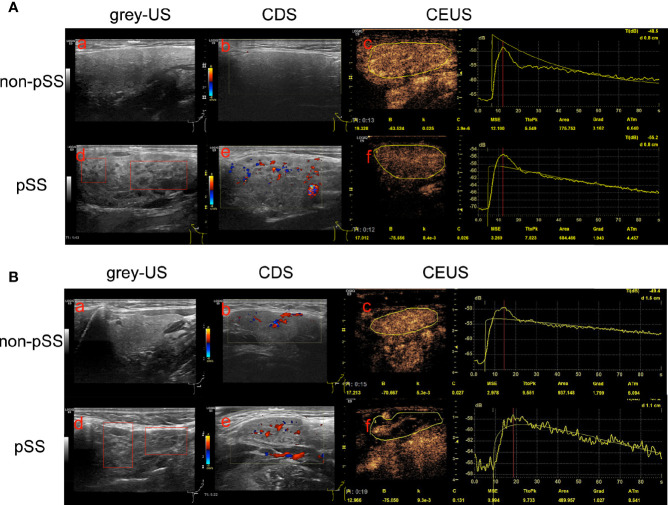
Multimodal ultrasonographic imaging characteristics of the parotid and submandibular glands between the primary Sjögren’s syndrome (pSS) and non-pSS subgroups. (A) Parotid glands: (a) homogeneous parenchyma without anechoic or hypoechoic areas in the non-pSS cohort; (d) diffuse inhomogeneity with anechoic or hypoechoic areas in pSS patients; CDS identified abundant hypervascularization in pSS cohorts (e) with poor vascularization in non-pSS groups (b); (c, f) No echo-free areas and delay TtoP in the parotid glands of pSS compared with non-pSS cohorts. (B) Submandibular glands: (a) homogeneous parenchyma without anechoic or hypoechoic areas in the non-pSS cohort; (d) diffuse inhomogeneity with anechoic or hypoechoic areas in pSS patients; (b, e) abundant hypervascularization detected in both pSS and non-pSS patients; (c, f) massive echo-free areas without contrast enhancement and significant delay TtoP after contrast media injection in pSS patients compared with non-pSS cohorts. US, Ultrasonography; CDS, Color Doppler sonography; CEUS, Contrast-enhanced ultrasonography; pSS, primary Sjögren’s syndrome.
